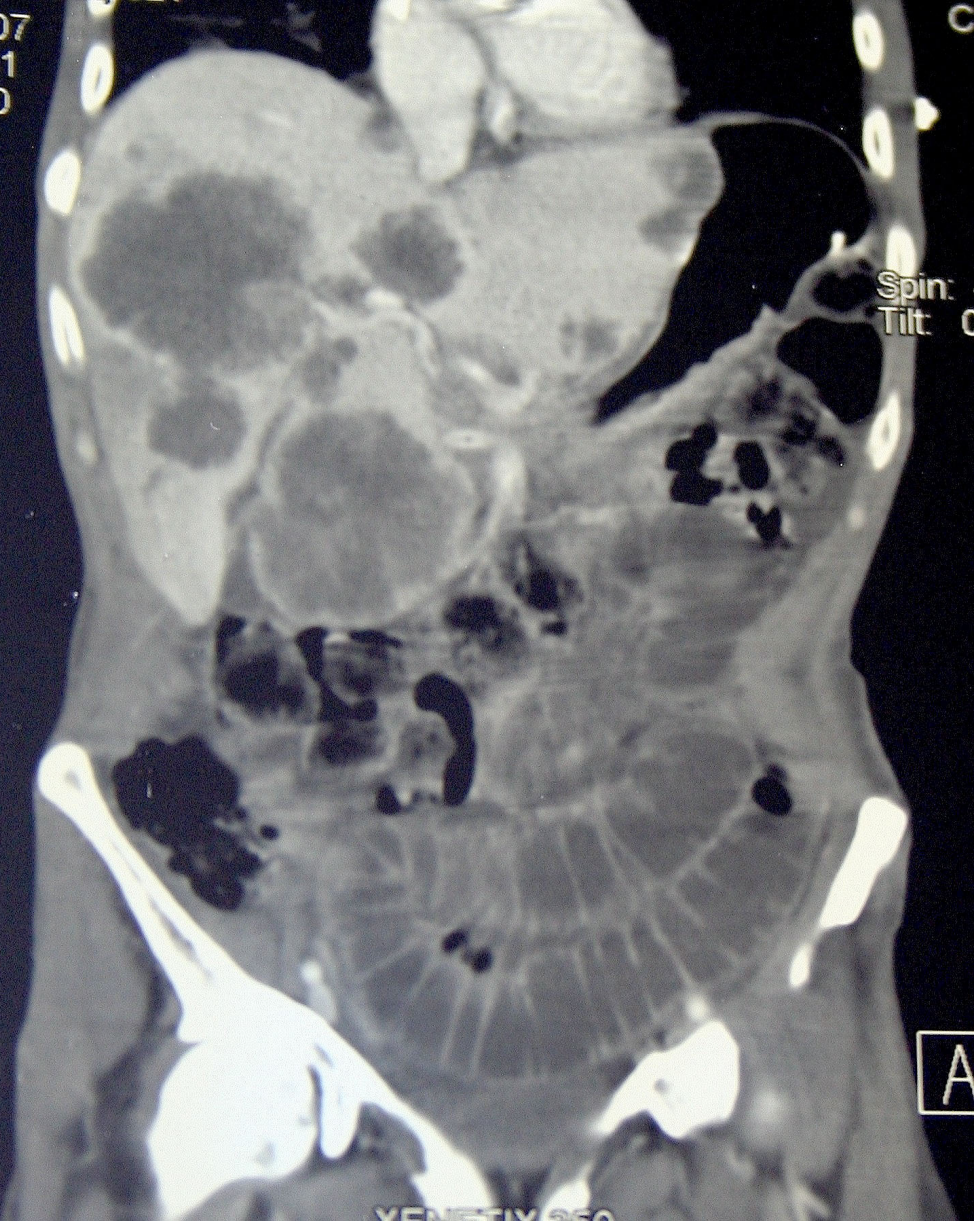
Abdominal CT scan performed before surgery shows both mechanical bowel obstruction and diffuse liver focal lesions due to advanced metastatic disease.
